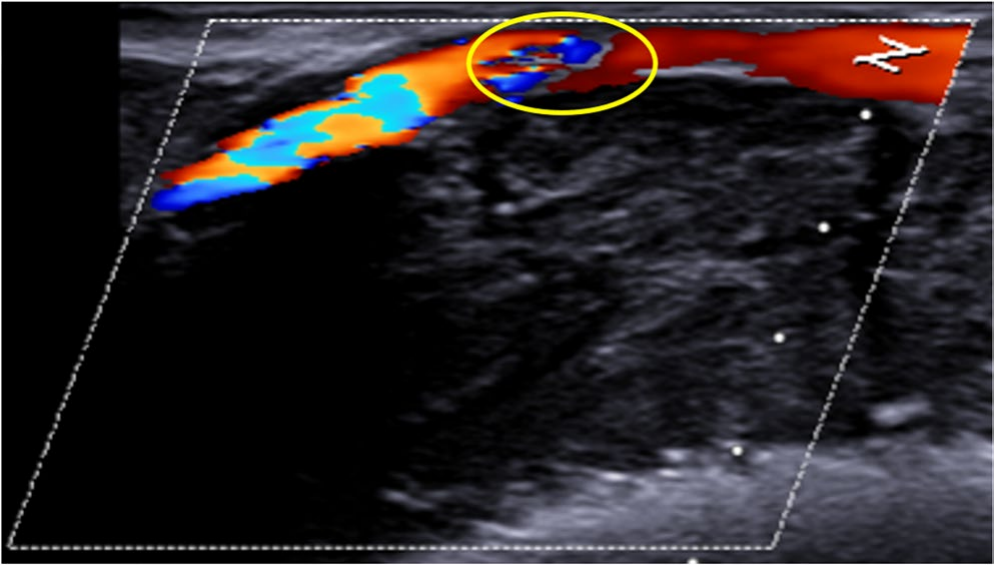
Longitudinal scan. Color Doppler ultrasound revealed turbulent flow within the segment obstructed by the atherosclerotic plaque (yellow oval) as well as in the downstream vascular segment
